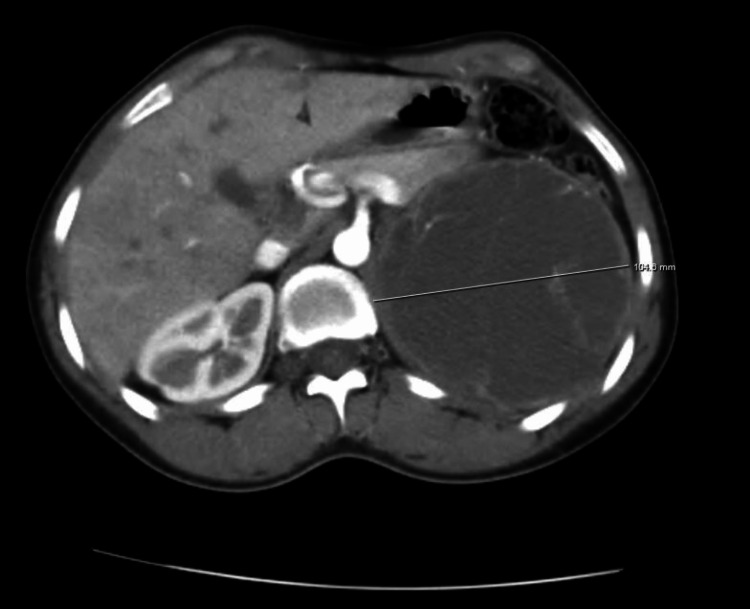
Axial section of an abdominal CT scan with IV contrast showing a 10.4 cm long cystic left adrenal mass containing fine contrast-enhanced septas and displacing the surrounding structures.
